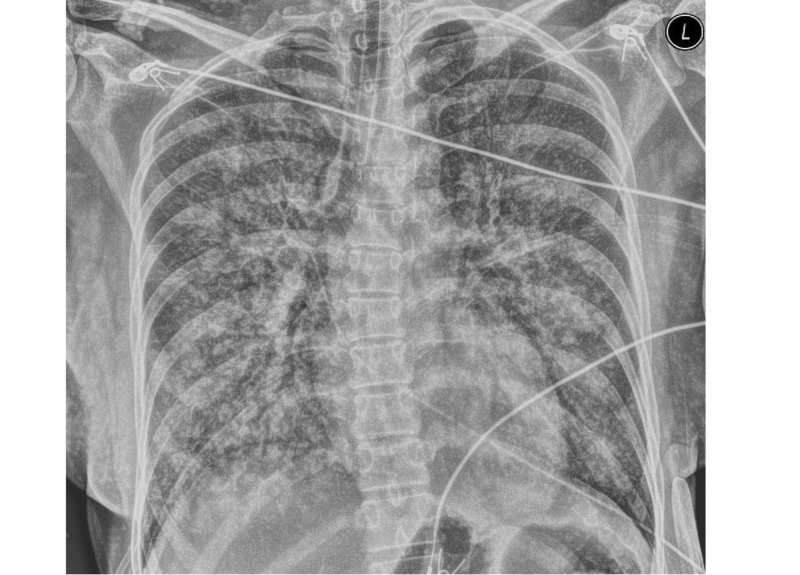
Chest X-ray A chest X-ray showing diffuse infiltrates consistent with cardiopulmonary edema or acute respiratory distress syndrome.
